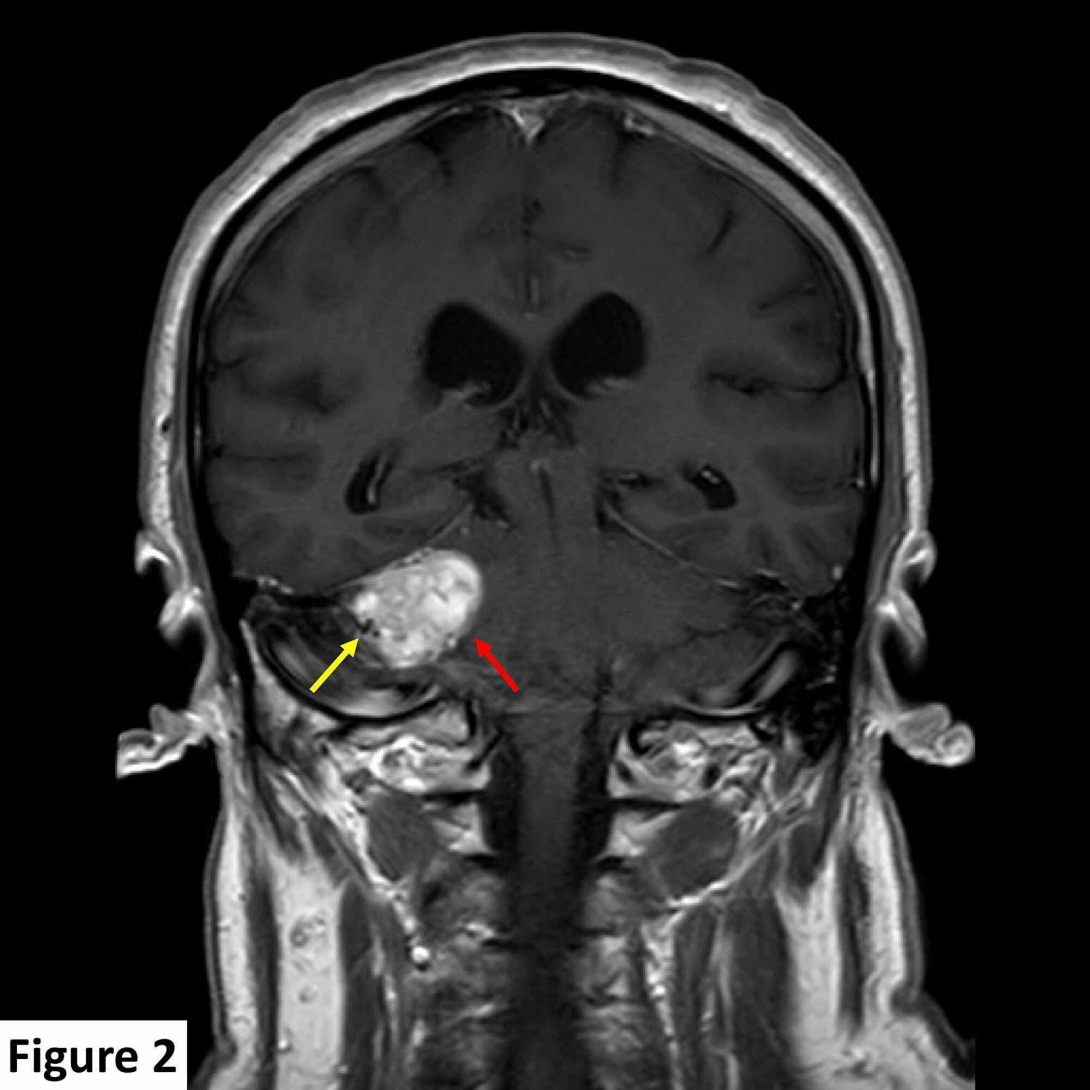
MRI scan at presentation. Coronal T1 contrast-enhanced image shows avid lesional enhancement (red arrow) and vascular flow void at its posterolateral aspect (yellow arrow). MRI, magnetic resonance imaging
